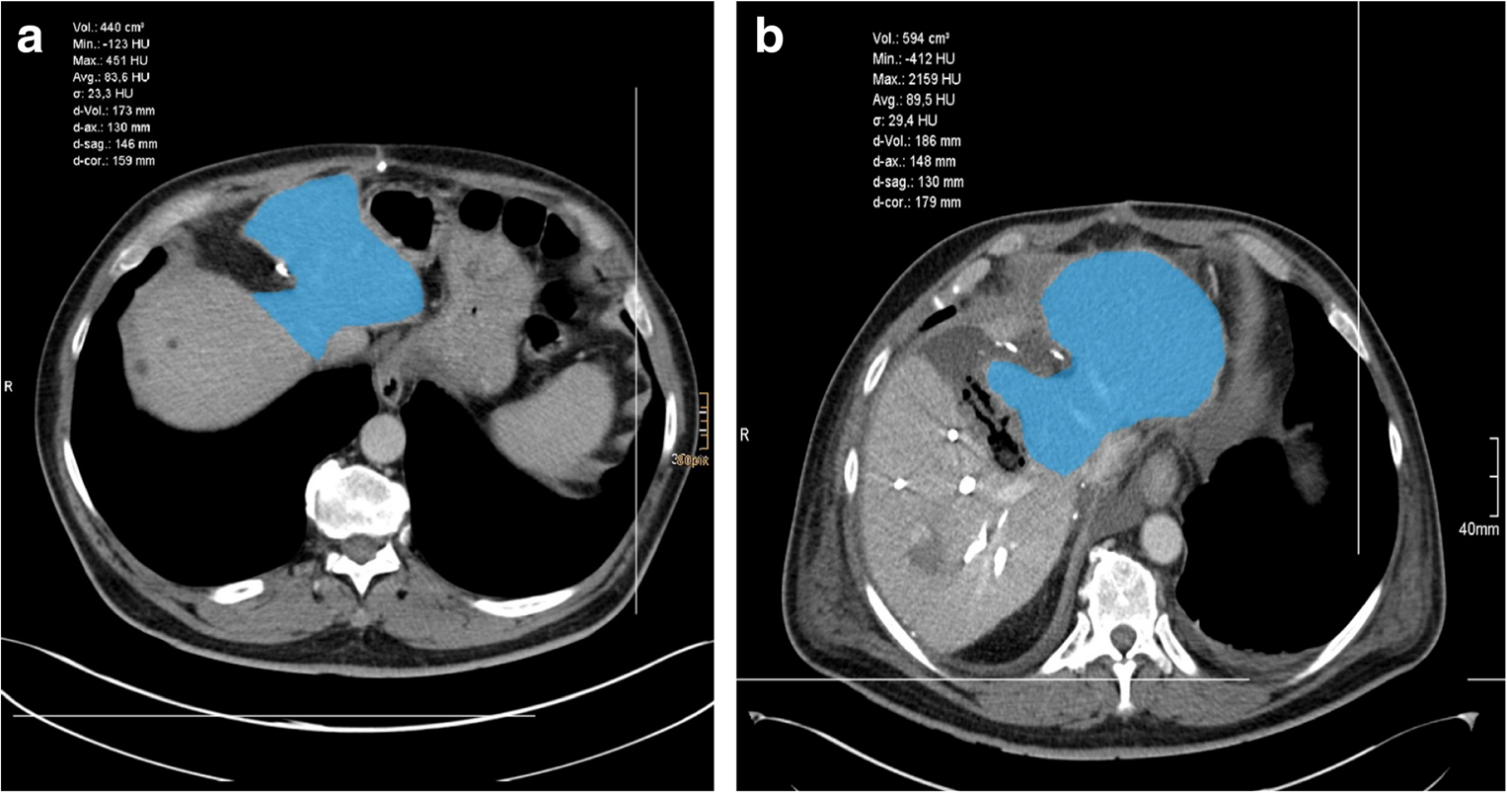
CT-based volumetry of the future liver remnant before (a) and 7 days after ALPSS step 1 (17.09.2019) (b)
